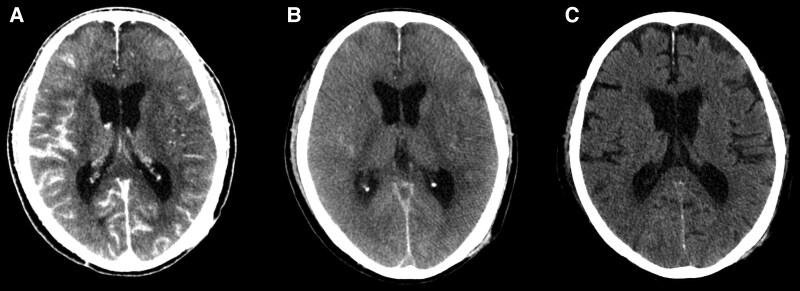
Plain head computed tomography on Days 0 (*A*), 2 (*B*), and 4 (*C*).
